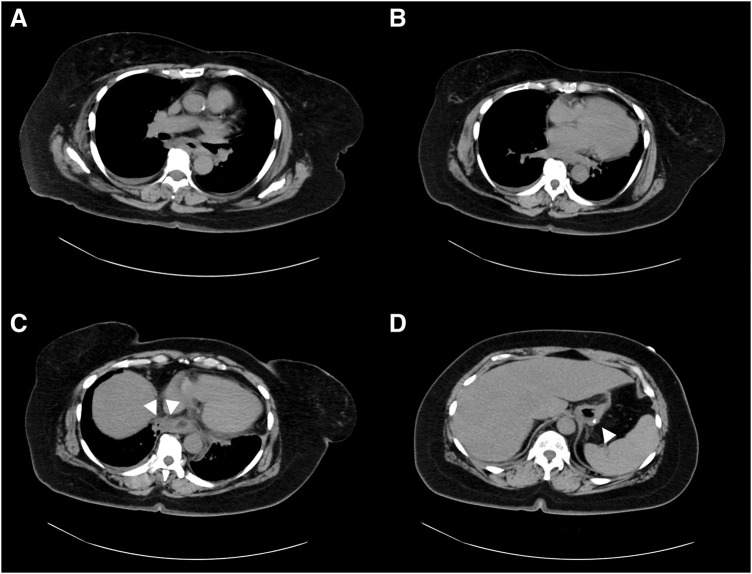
CT scan serial transverse planes from tracheal bifurcation to spleen at admission. (A) At the tracheal bifurcation level, no obvious abnormalities were noted. (B) At the right atrium level, no apparent abnormalities were noted. (C) At the EGJ level, the esophageal wall was slightly thickened circumferentially, and a small amount of fluid collection was noted on the right side of the esophagus (white triangles). (D) The upper portion of the linear staple was visible in the spleen. No free air or fluid was collected (white triangle). EGJ, esophagogastric junction
